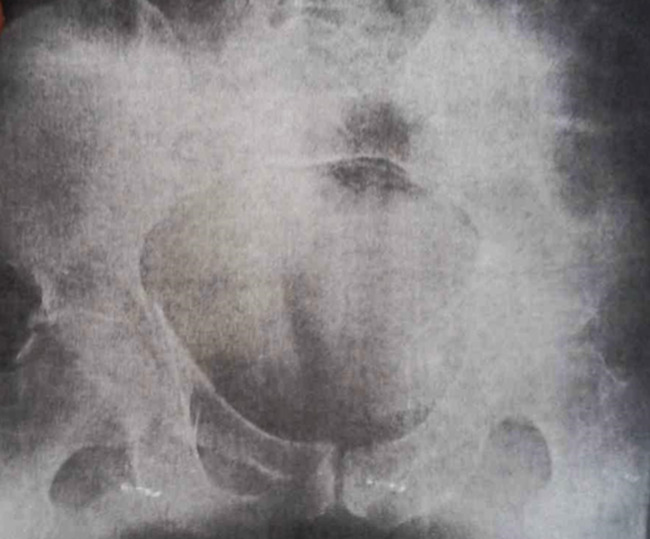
standard radiograph of the pelvis after reduction
